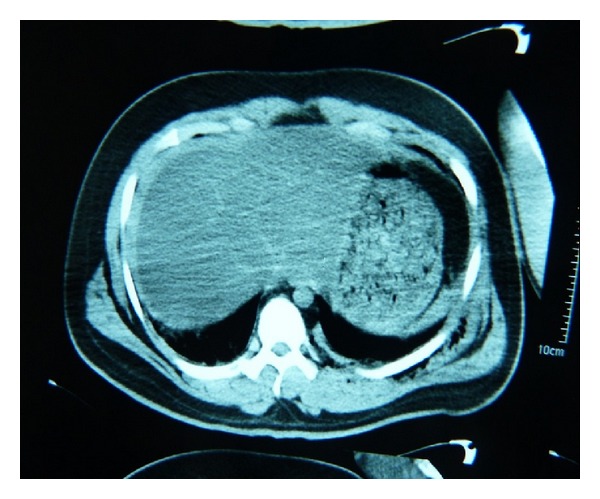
Abdomen CT image of the patient.
